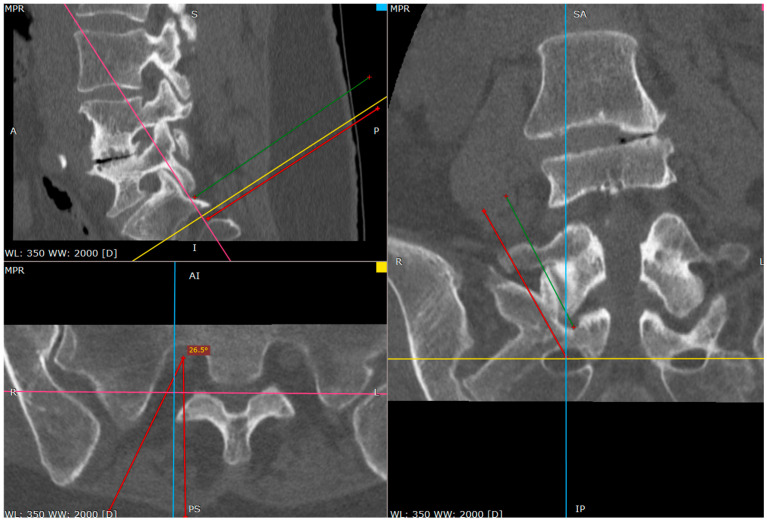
Approach angle and needle trajectory to S1 root (CT tri-plane reconstruction).
